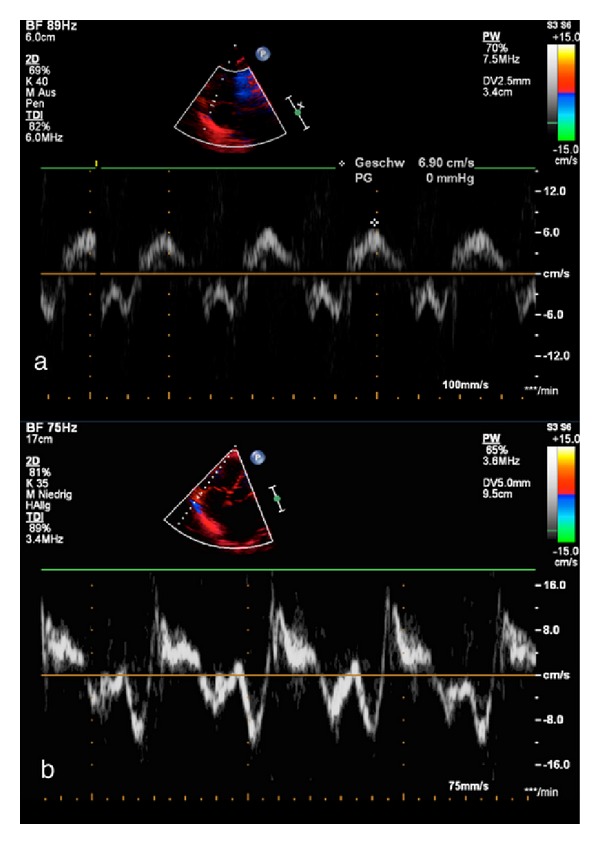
Apical 4-chamber view. The white broken line indicates M-mode cursor placement at the tricuspid lateral annulus. Representative image of the tricuspid annular peak systolic velocity (TAPSV) in a neonate (Figure 1(a)), and in a 15-year-old adolescent (Figure 1(b)), respectively, with normal right and left ventricular function.
